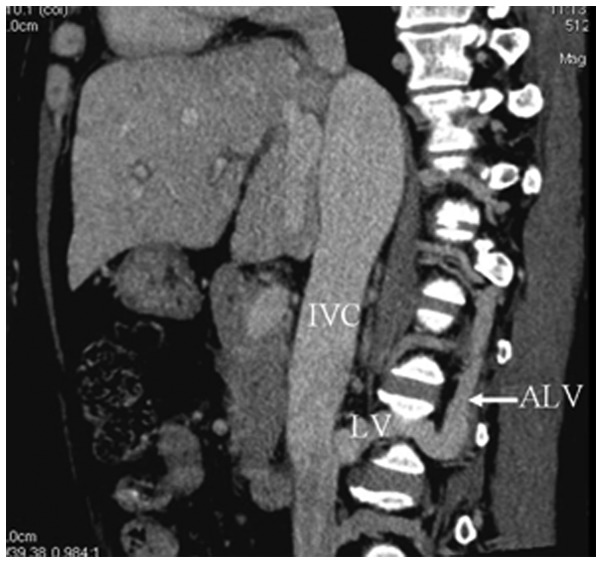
IVC-LV-ALV-azygos/hemiazygos vein collaterals. Computed tomography angiography shows membranous occlusion of the IVC. Venous flow within the IVC reverses to the LV and then continues through the ALVs, anastomosing with the azygos/hemiazygos vein system. IVC, inferior vena cava; LV, lumbar vein; ALV, ascending LV.
